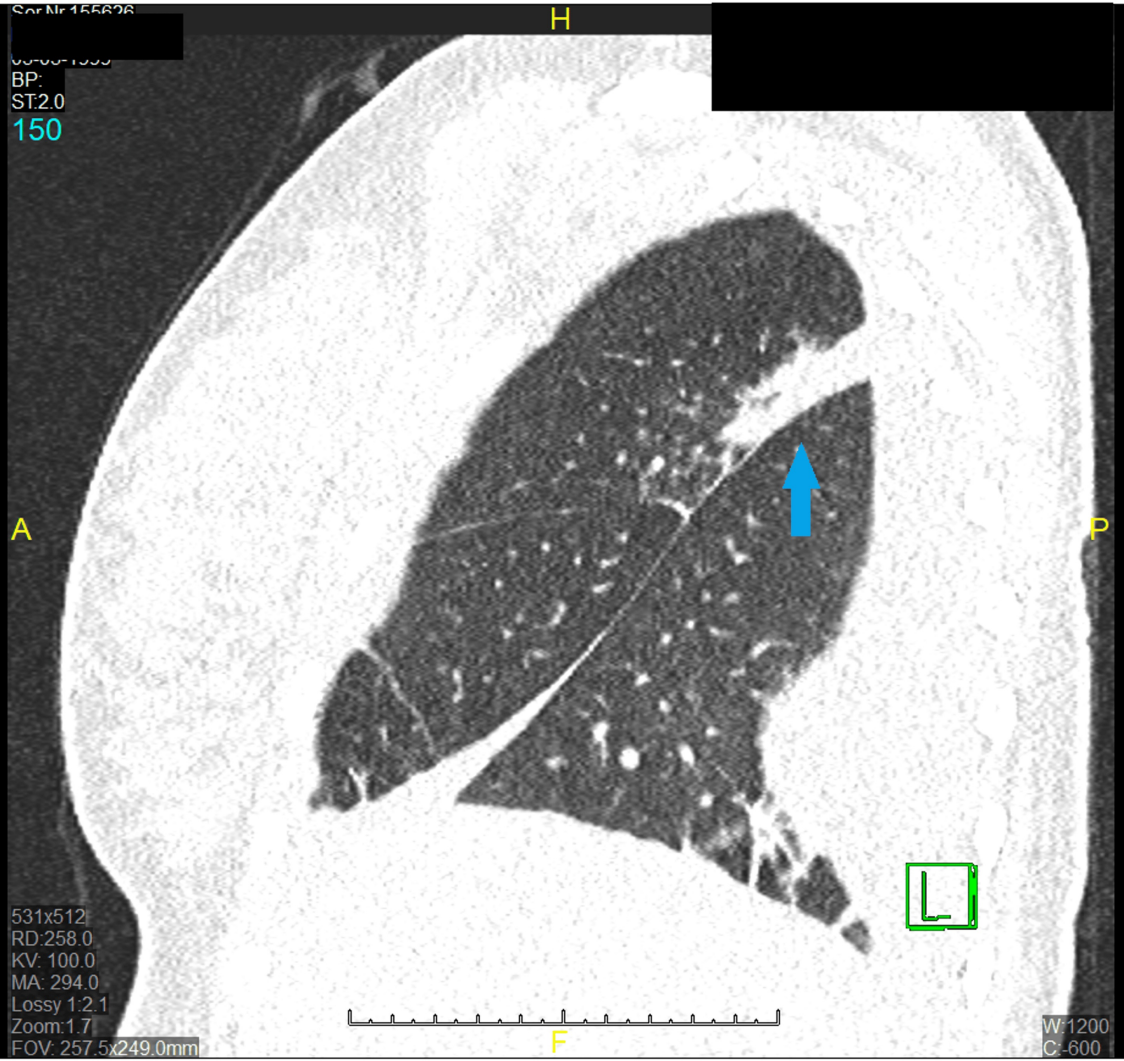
Low-dose CT of the lungs showing pulmonary consolidation A sagittal plane view of the CT scan showing pulmonary consolidation on the right side (blue arrow).
